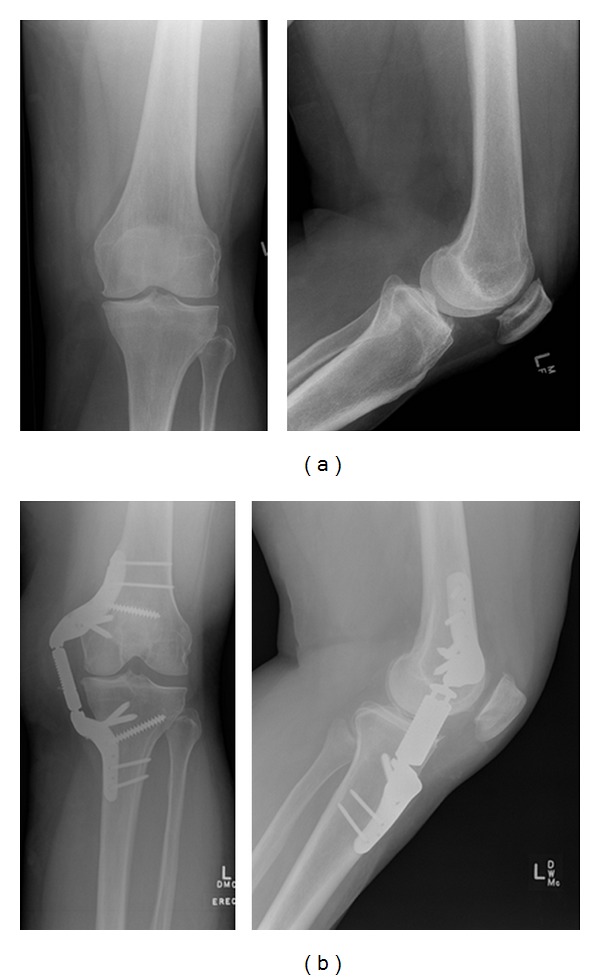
Anterior (left) and medial (right) radiographic view of the left knee at pretreatment (a) and at 3 years demonstrating the KineSpring System *in situ* (b).
